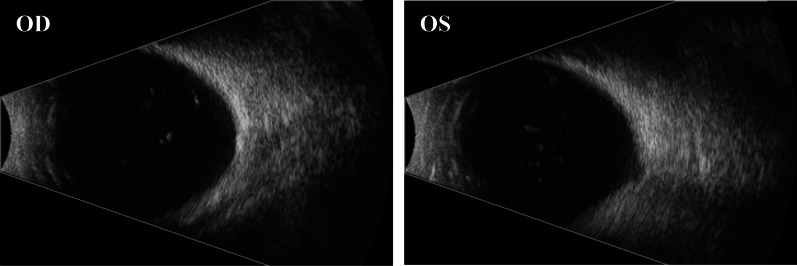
Binocular B-ultrasound examination. The prolongations of the axial axis were 28.73 mm in the right eye (left panel) and 28.38 mm in the left eye (right panel)
